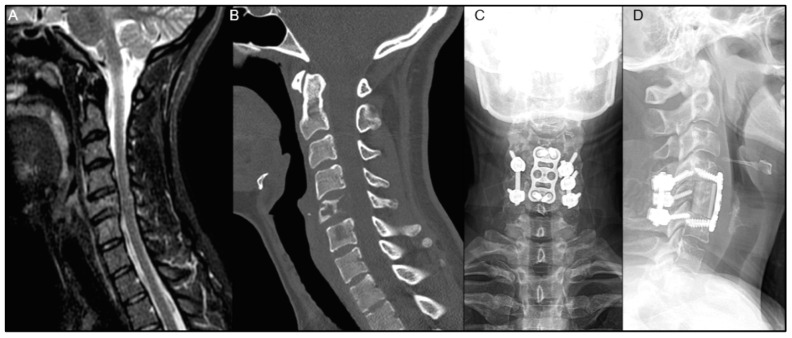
(A–D) A 26-year-old female presented with severe mechanical neck pain for several months and was found to have a C5 lytic lesion affecting 70% of the vertebral body as seen on preoperative sagittal T2-weighted MRI (A) and CT scan (B). The patient also had a left C6 radiculopathy, along with numbness and paresthesia, but with no reported weakness. She underwent separation surgery with a C5 corpectomy and C4–C6 anterior fusion with fibular allograft, as well as a posterior C4–C6 instrumented fusion, as seen on postoperative posteroanterior (C) and lateral (D) X-rays. The patient received SBRT for 2 weeks postoperatively and did not encounter any postoperative wound complications.
